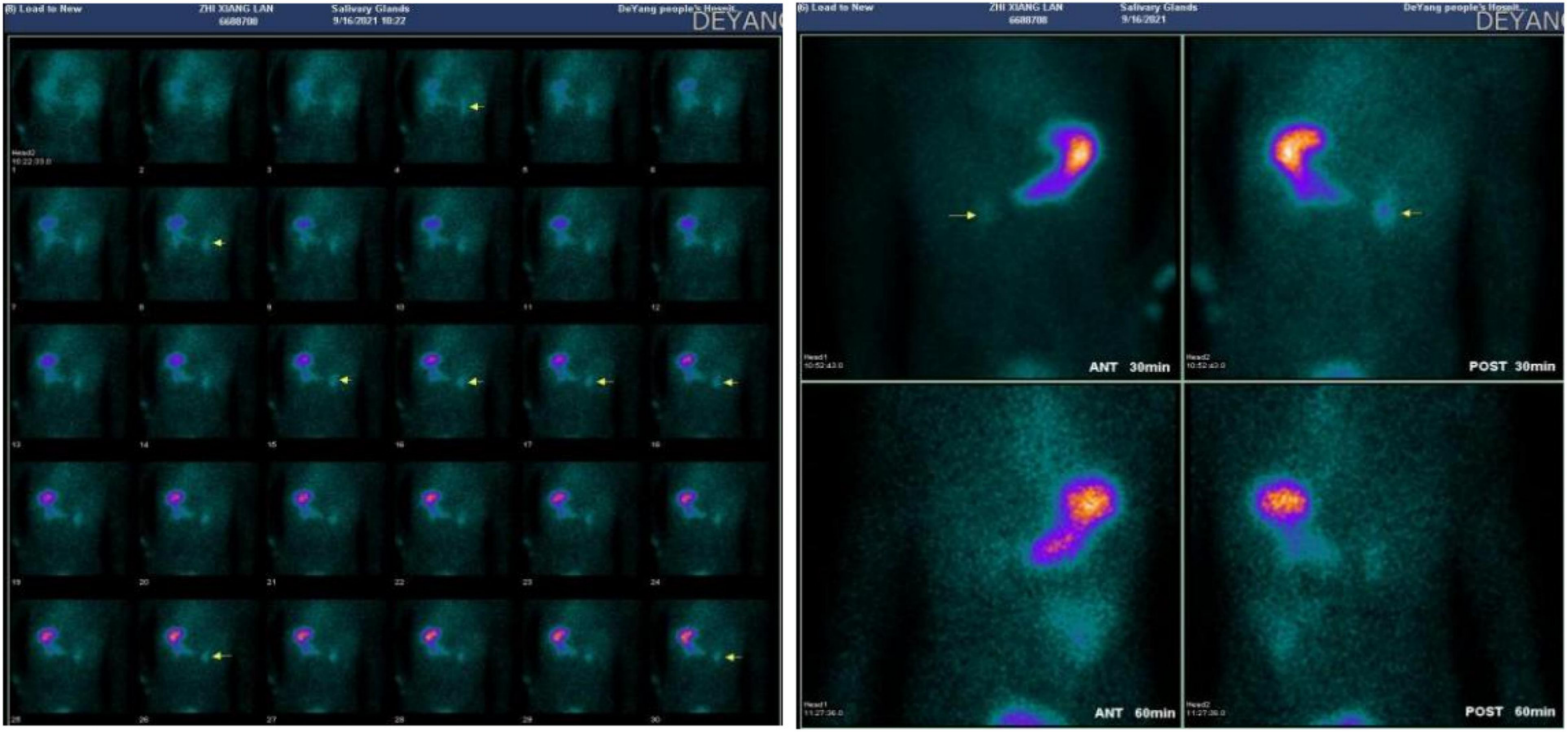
99mTcO4− scintigraphy revealing ectopic gastric mucosa in the second part of the duodenum.
